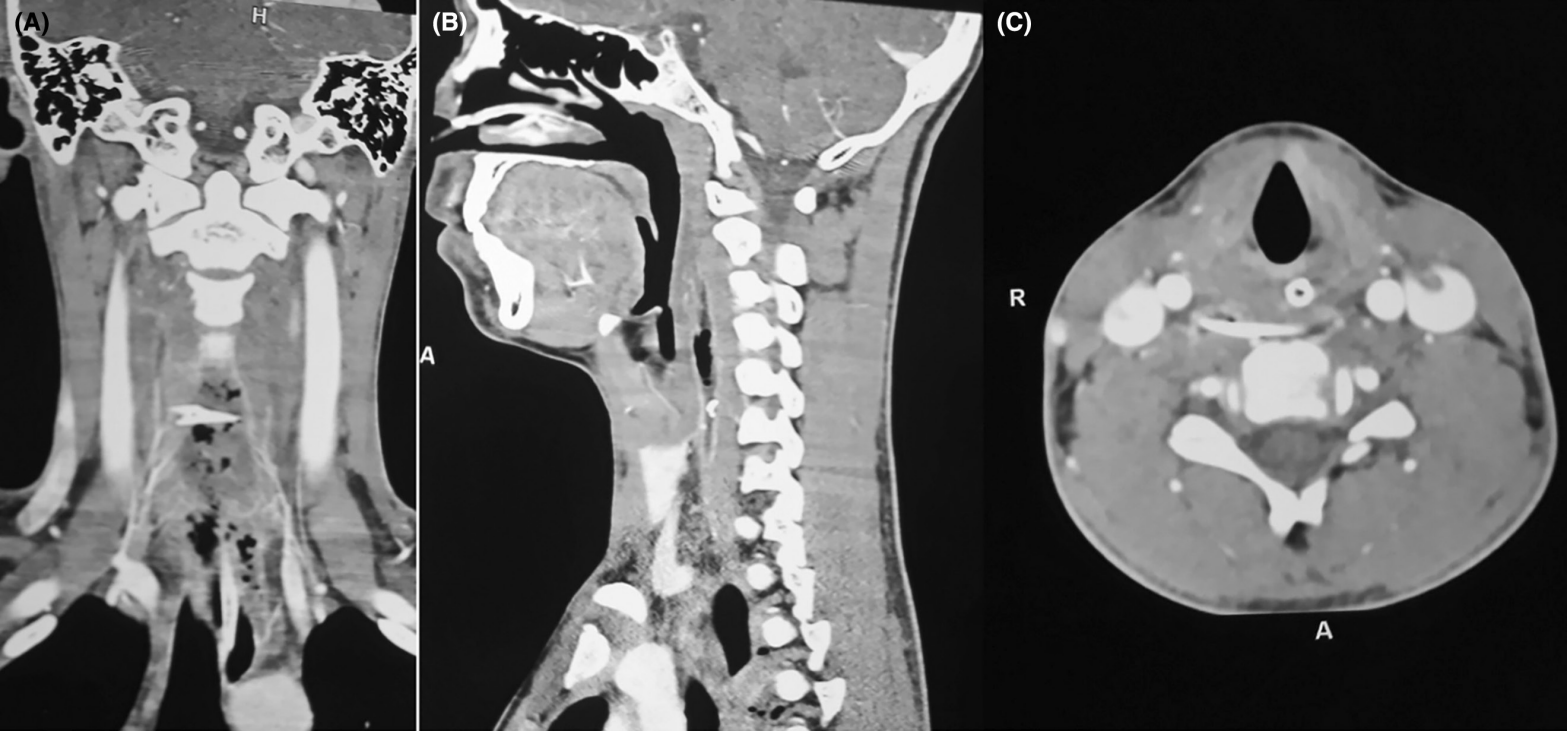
(A) CT coronal‐linear horizontally oriented bone density shadow; (B) Ct sagittal vertically elongated hypodense area in front of C3‐C6 and hyperdense shadow in front of C5‐C6; (C) CT axial horizontally placed foreign body at C5.
